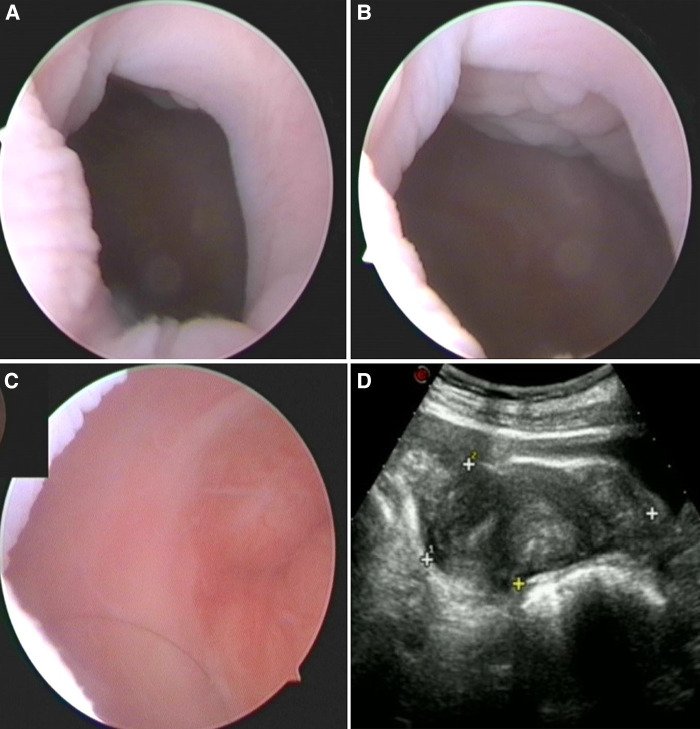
Outpatient hysteroscopic and ultrasonic images 3 months after operation. (A,B) Hysteroscopy showed that the surgical wound healed well. (C) The cervix could be observed. (D) A pelvic ultrasound showed no abnormalities.
